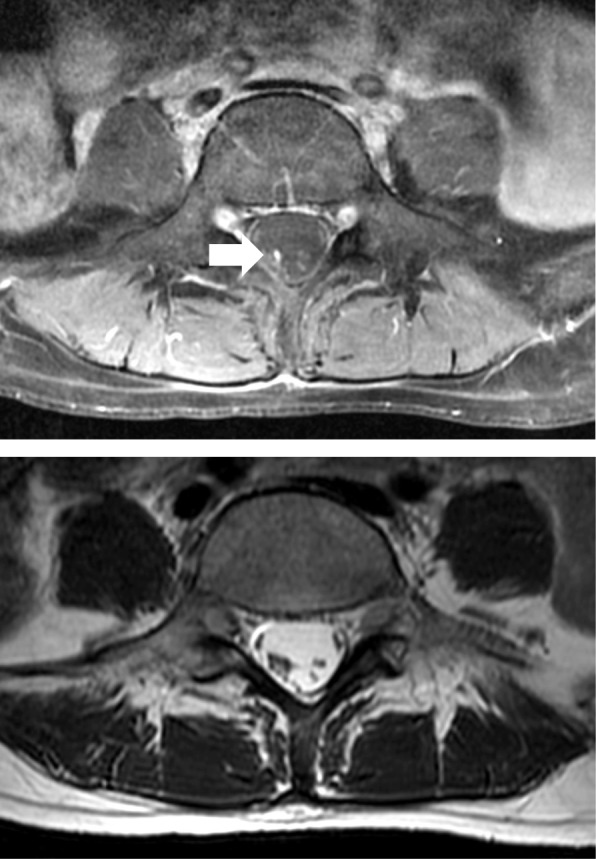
An axial gadolinium-enhanced T1-weighted image at the level of L5 and a T2-weighted image at the same level. These images show clumpy cauda equina fibers within dura mater. White arrow indicates a cauda equina aggregated posteriorly, with identifiable blood in a white dot. The clumped, asymmetric appearance of cauda equina fibers and contrast enhancement indicates the existence of inflammation
